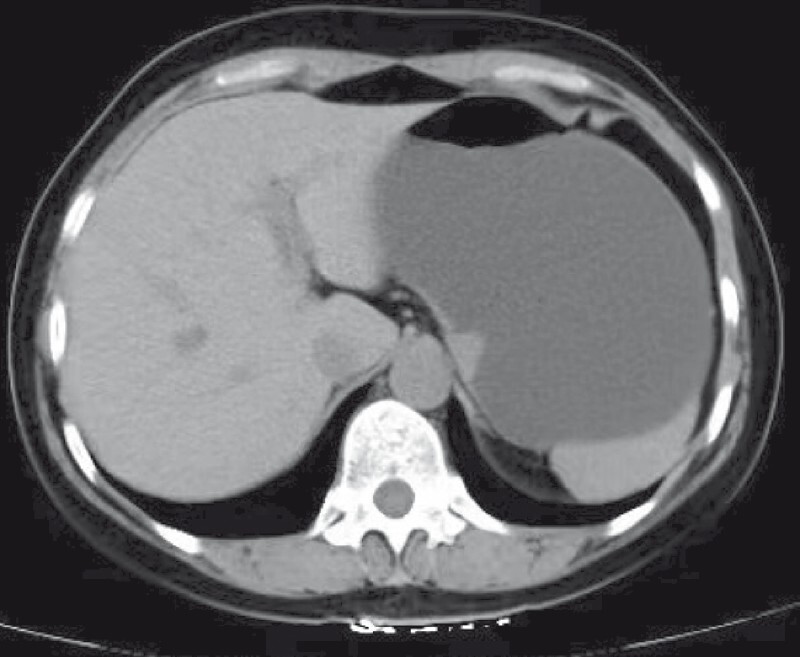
Computed tomography image showing a stromal tumor (about 3 × 2 cm) in the upper gastric body.
